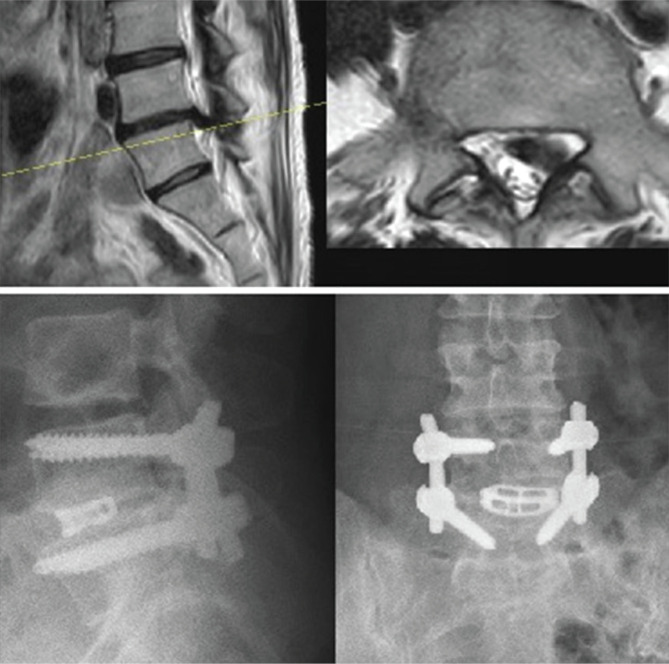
Preoperative magnetic resonance images and postoperative X-ray images.
